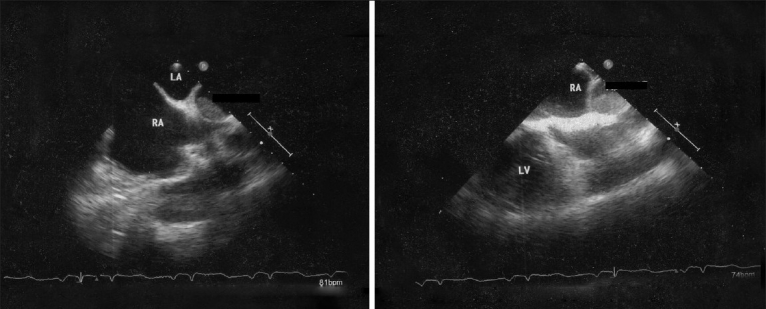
Transesophageal echocardiography in modified short-axis views. LA=Left atrium; RA=Right atrium; LV=Left ventricle
